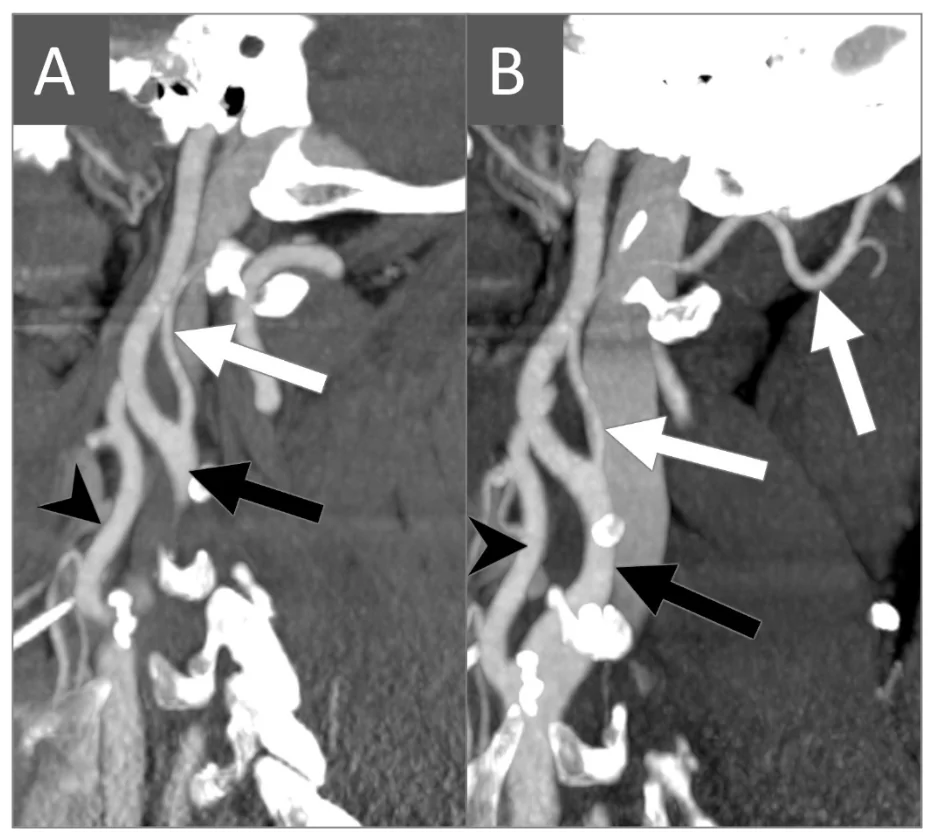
Occipital artery originating from the left ICA. Sagittal CTA MIP images (A,B) show an aberrant origin of the occipital artery (white arrows) from the ICA (black arrows). Black arrowhead: ECA.
